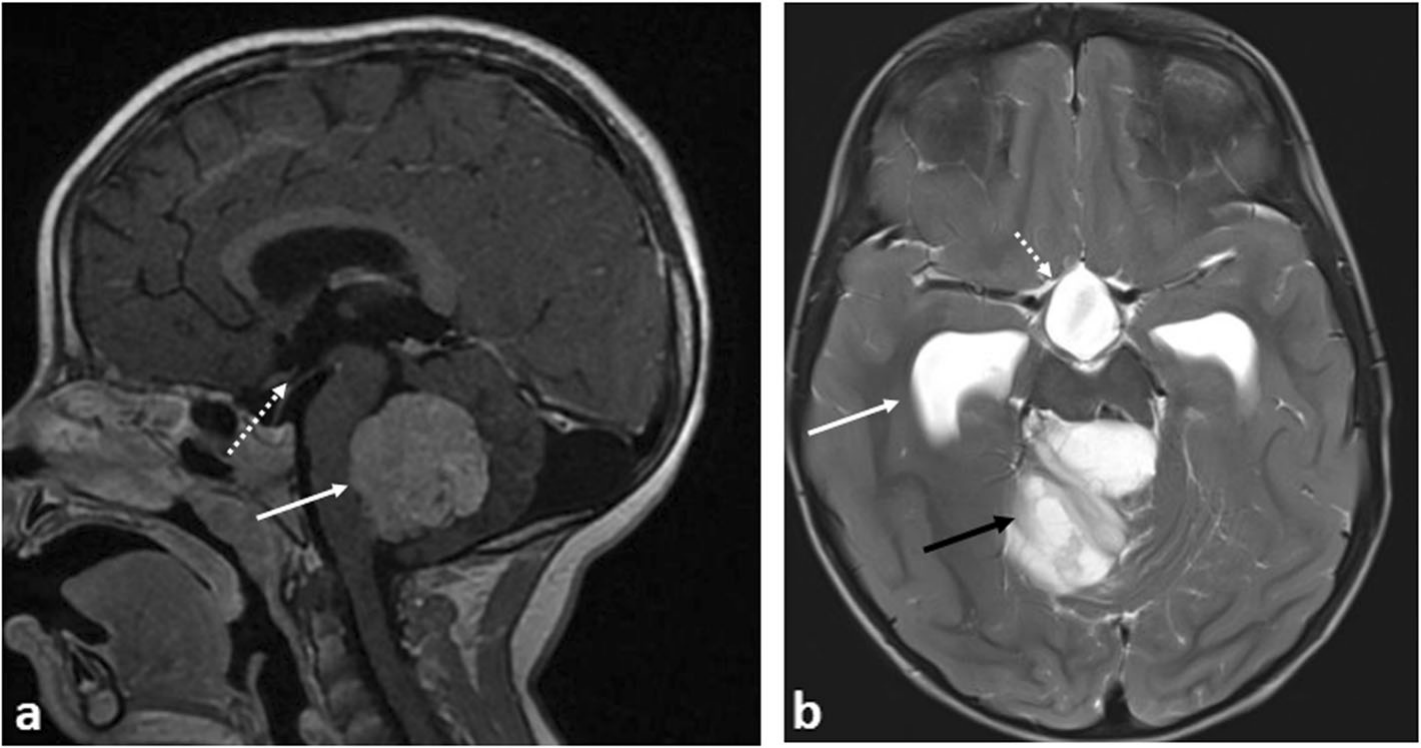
Three examples of causes of obstructive hydrocephalus in different patients due to different intracranial tumours. a Sagittal post-contrast T1-weighted MRI image showing a medulloblastoma in the fourth ventricle with mass effect on the brainstem (solid white arrow). There are no early signs of hydrocephalus—the floor of the third ventricle is not bulging inferiorly (dashed white arrow)—despite the compression of the brainstem. b Axial T2-weighted MRI image in patient with a pilocytic astrocytoma in the posterior fossa (black arrow) with signs of hydrocephalus and dilatation of the temporal horns (solid white arrow) of the lateral ventricles and inferior dilatation of the third ventricle (dashed white arrow)
